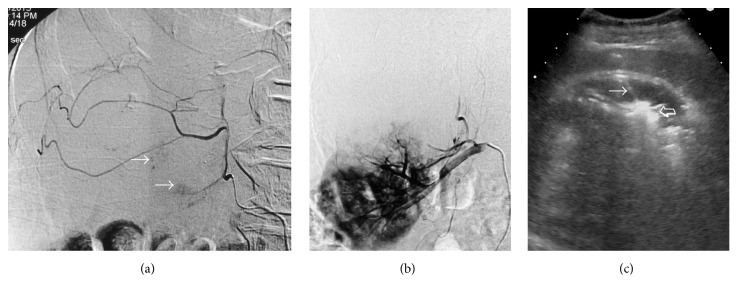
Representative images from the interventions. (a) Digital subtraction angiography (DSA) image after superselective catheterization of the tumor feeders shows relatively few and thin tumoral arteries and a limited tumor blush (arrows). (b) DSA image after embolization shows devascularization of the tumor with preservation of the renal enhancement. (c) Coronal oblique sonographic image during ablation shows the electrode (arrow), which has been advanced into the soft-tissue part of the tumor. Strong echoes (open arrow) caused by tissue vaporization are noted around the electrode tip.
